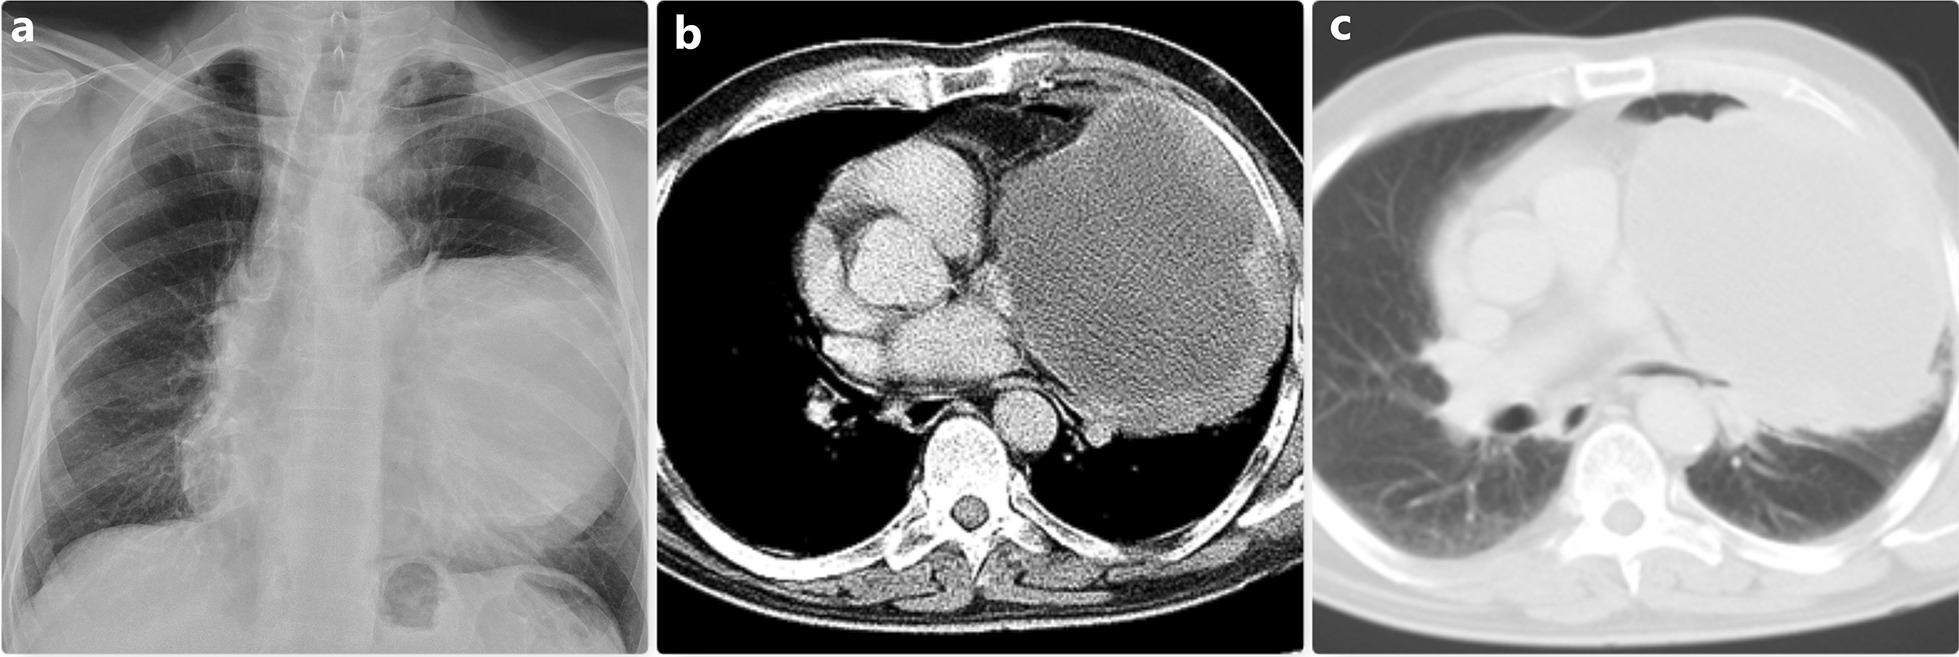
The left-sided cavity was seen in the chest radiogram (a) On thorax CT, a giant cavitary lesion pushing the mediastinum to the opposite side is observed (b and c) In this patient, the diagnosis was made as large cell carcinoma
